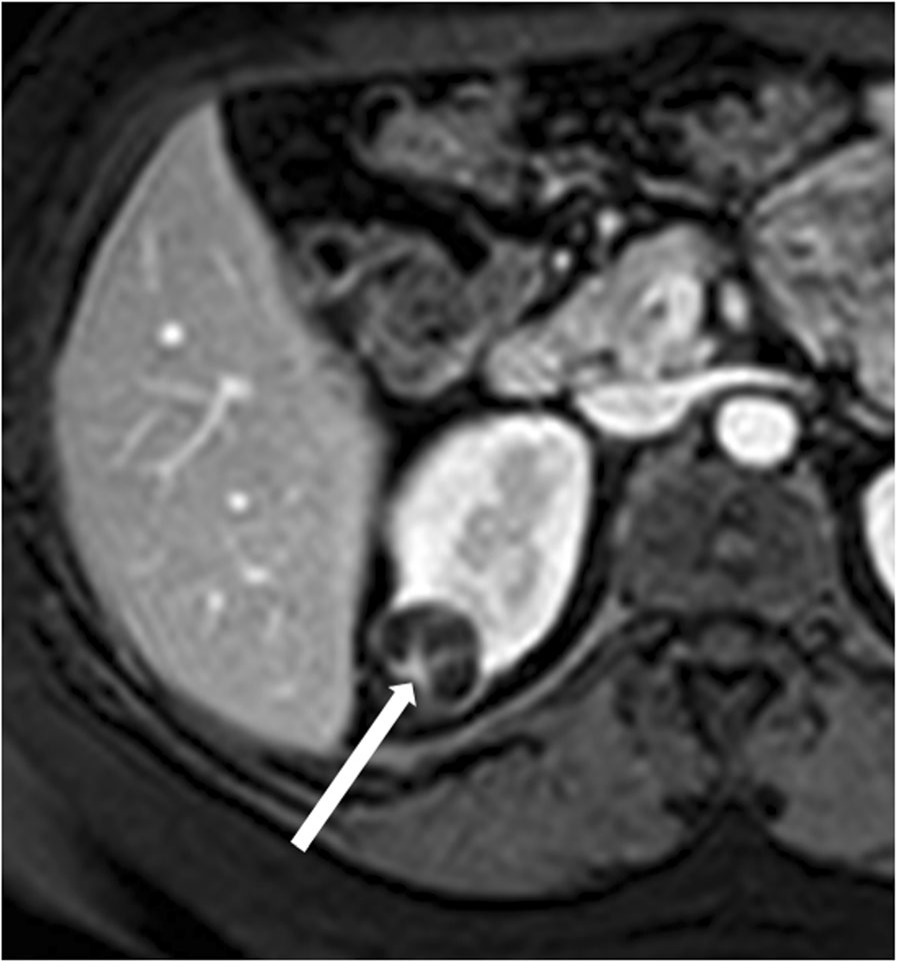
Bosniak category III renal cyst. Axial contrast-enhanced T1-weighted MR image shows a lesion with thick, enhancing wall and septa (arrow)
